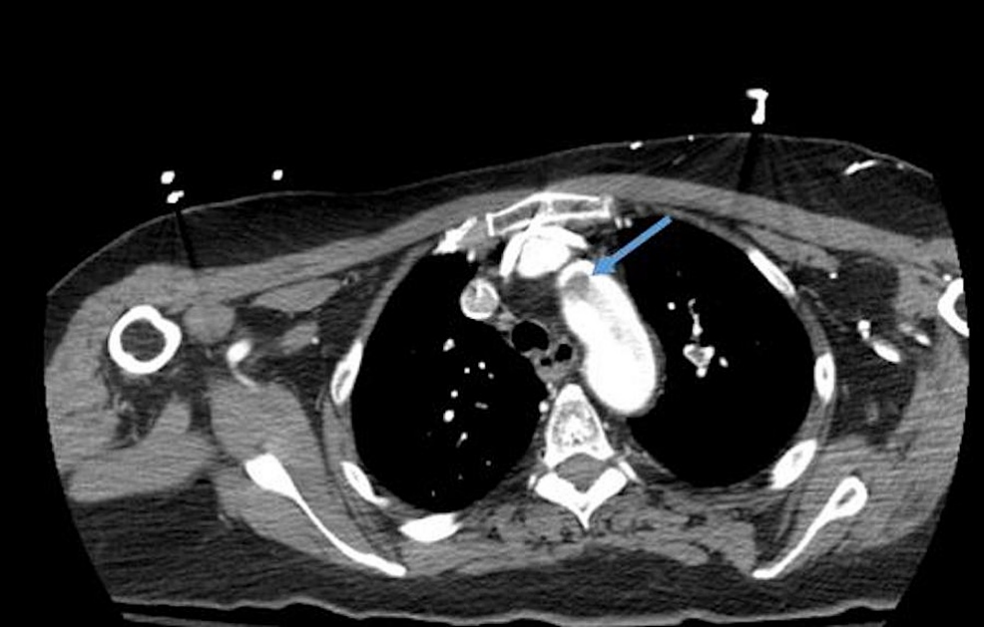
Computed tomography angiography (CTA) - blue arrow showing thrombus in aortic arch
